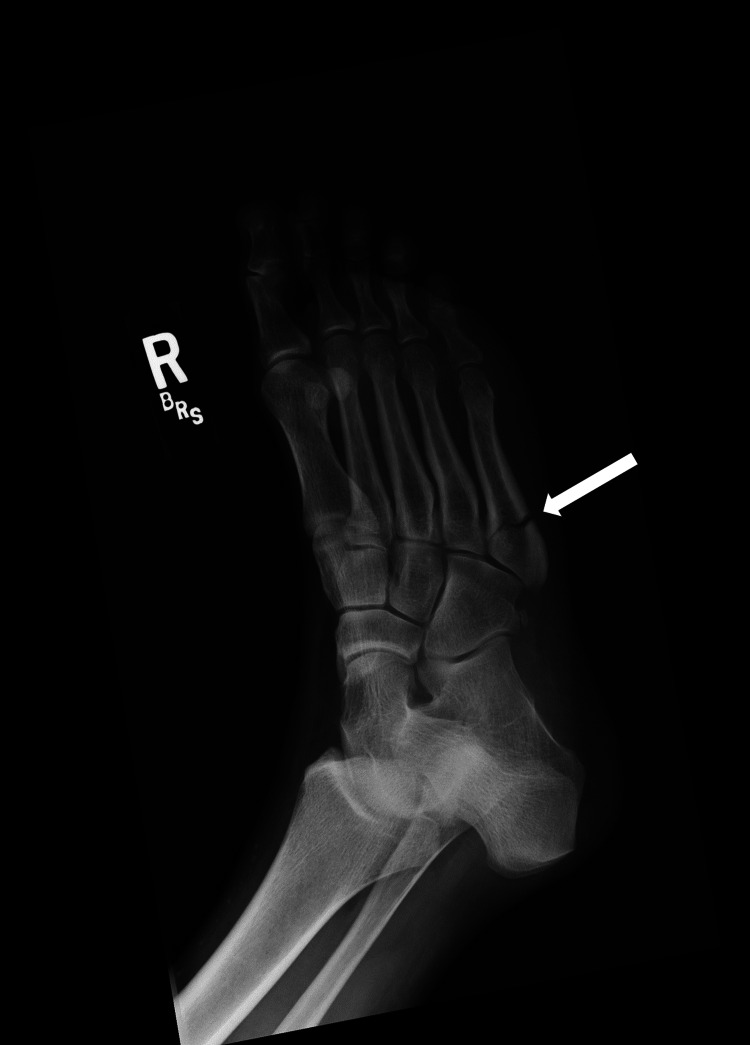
Oblique radiograph of the right foot demonstrating a mildly displaced transverse fracture of the proximal fifth metatarsal (arrow).
